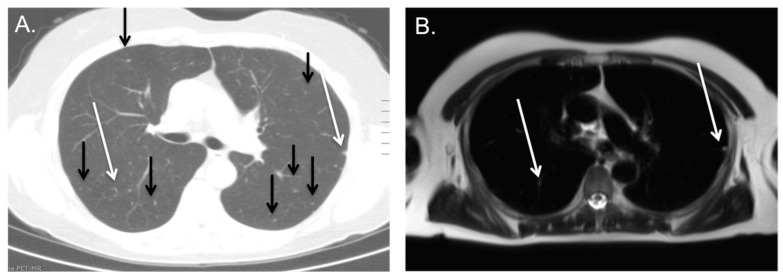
Poor detection of indeterminate Nodules on PET/MRI; patient with a pancreatic carcinoma with many millimetric lung nodules on CT (A), of which only a few are visible on MRI (B, see white arrows on CT and MRI), but most could not be detected due to their small size (see black arrows on CT). Lung nodules remained stable in follow-up and were classified as benign.
